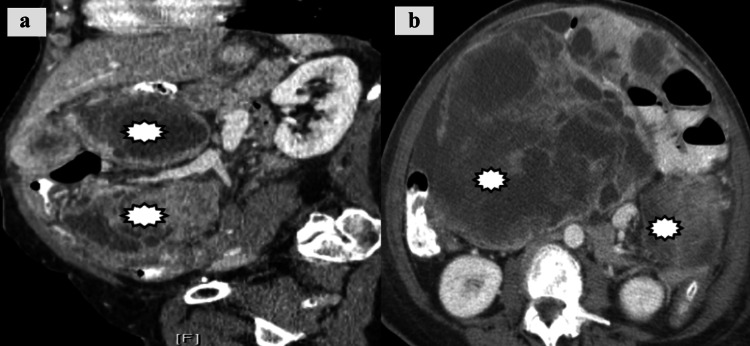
Peritoneal carcinomatosis. Heterogeneous necrotic peritoneal masses (white bursts) secondary to metastatic endometrial carcinoma seen on (a) sagittal and (b) axial on CT imaging.
